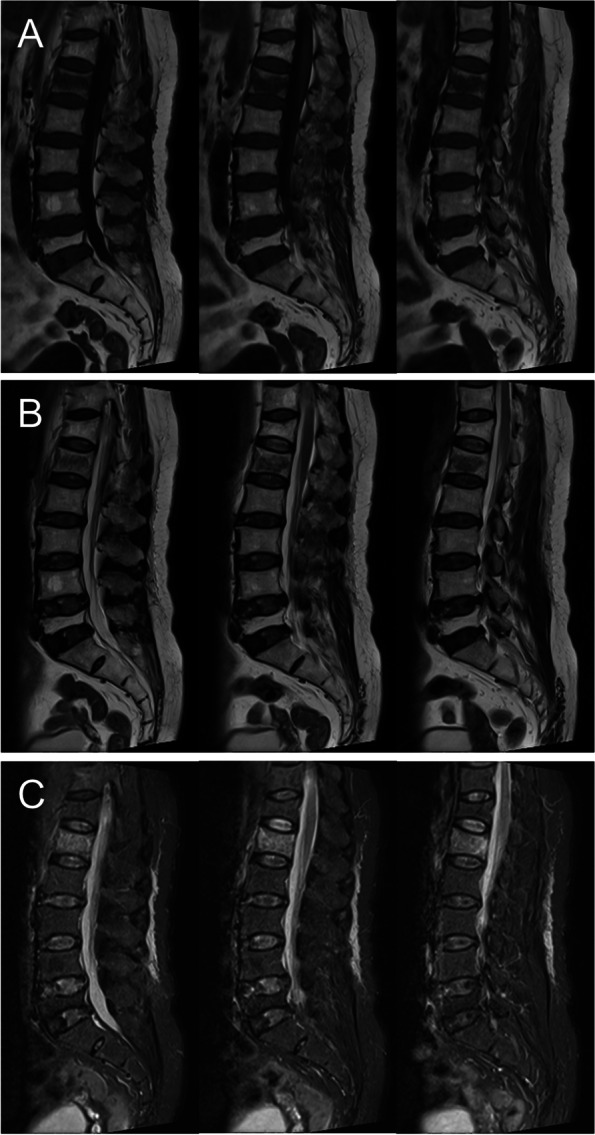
Osteoporotic vertebral fracture with severe fascia injury. Three most central slices of sagittal T1-weighted (A), T2-weighted (B) and T2-weighted fat suppression (C) MR images show L1 vertebral fracture with severe FI
